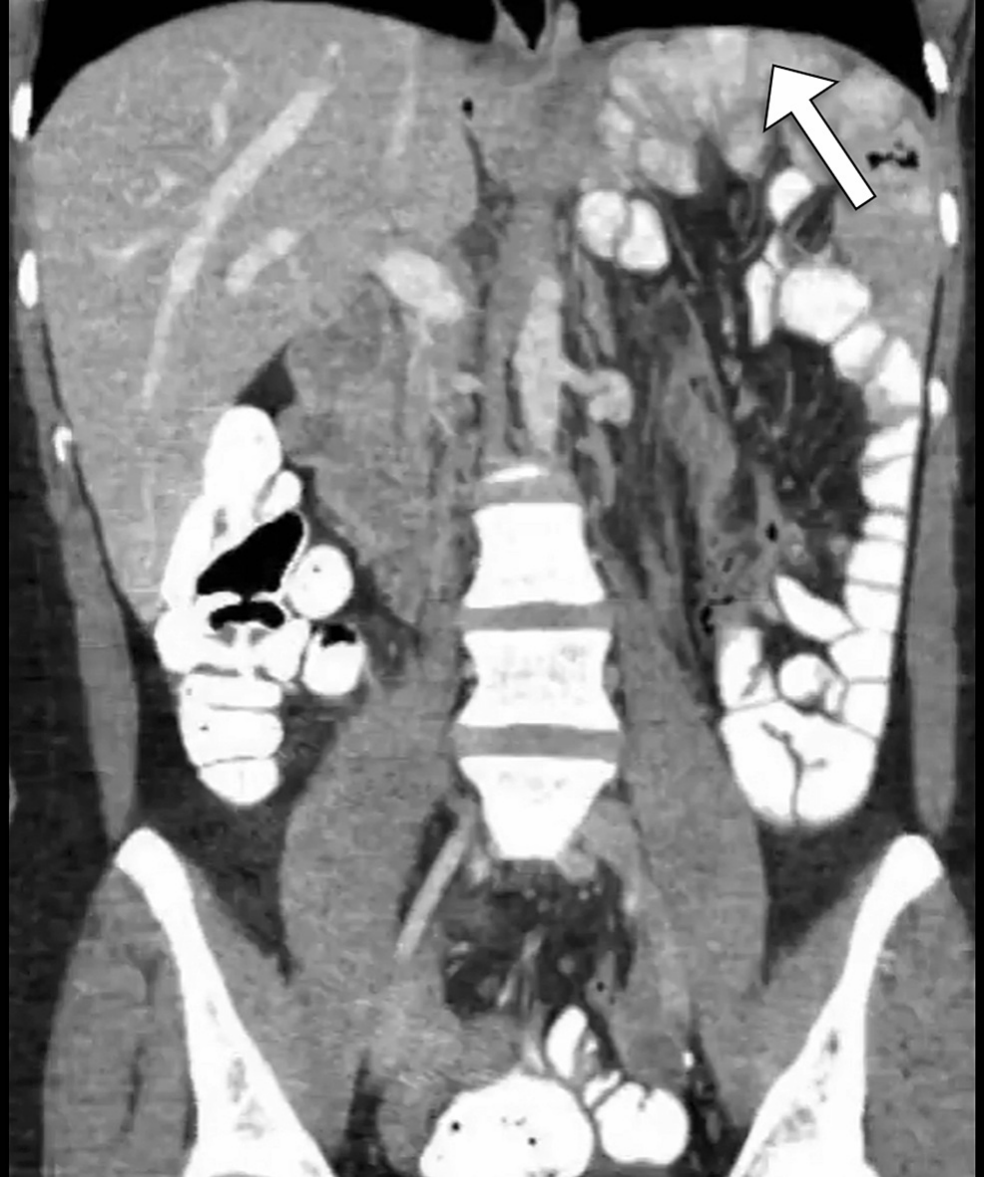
Coronal CT image of the abdomen shows the absence of the spleen in the upper quadrant of the abdomen (arrow).
